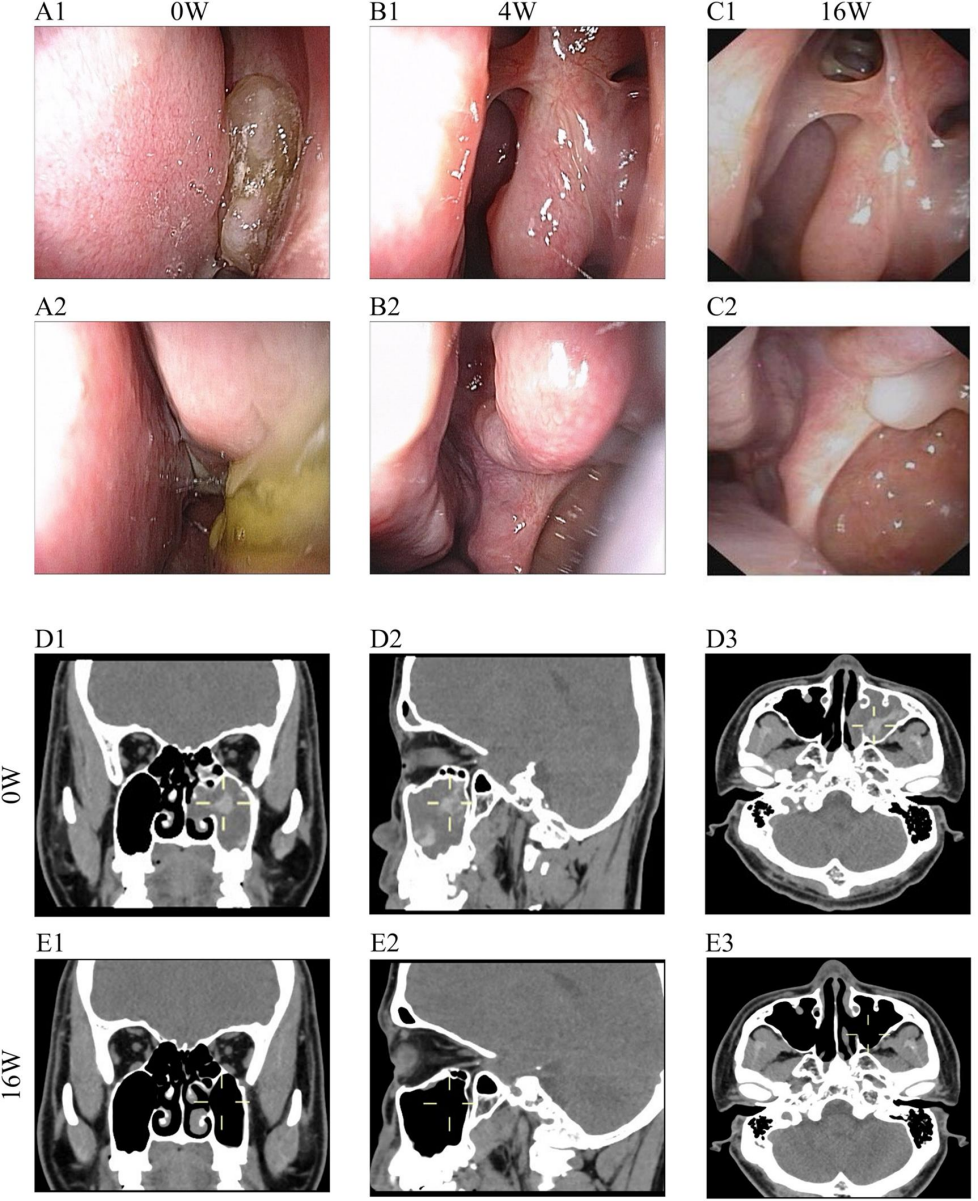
Imaging data of the case. (A–C) Comparison of endoscopic changes pre and post stapokibart therapy. (A1-A2) prior to treatment. (B1-B2) At 4-week follow-up after stapokibart therapy, endoscopic examination revealed complete resolution of the jam-like inspissated secretions in the left middle meatus. (C1-C2) 16 weeks after stapokibart therapy. (D) Pre-treatment CT scan of paranasal sinuses demonstrates unilateral, expansile lesions with heterogeneous opacities and noninvasive features. (E) 16 weeks after stapokibart therapy showed complete resolution of the inflammatory opacities in the left maxillary sinus and improvement of the ethmoid sinus mucosa.
